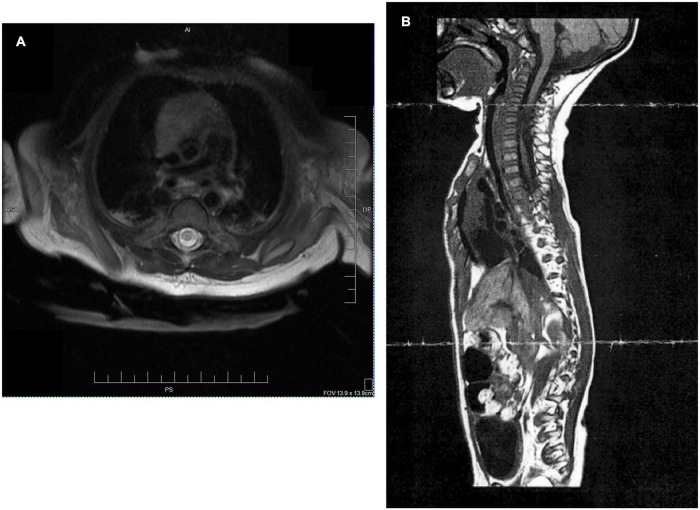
(A) Transaxial T2 sequence: MRI of cervical spine revealed cystic myelomalacia involving mid and lower upper thoracic spinal cord between inferior C4 and T1 levels at age 1 month. (B) MRI sagittal image at 11 months of age again shows cystic myelomalacia from C4-T1.
